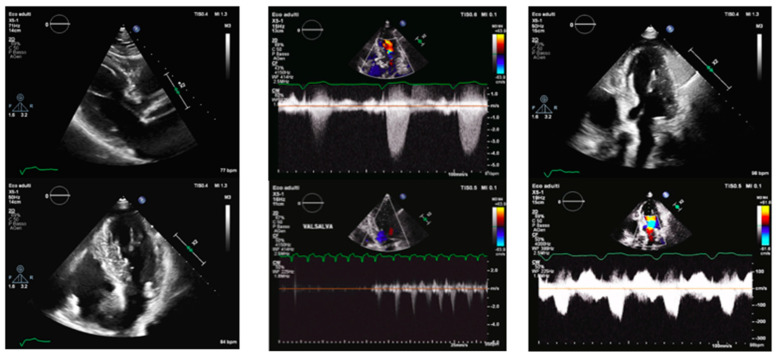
Transthoracic echocardiography of a 79-year-old woman before and after surgical aortic valve replacement and septal myectomy. Left panel. PLAX view (upper) and 4-chamber view (lower). IVS telediastolic diameter = 15 mm; LVEDVi 27 mL/m^2^. Central panel. Aortic valve peak velocity of 4.5 m/s (upper) with a mean gradient of 51 mmHg and dynamic LVOT gradient (lower) of 42 mmHg after the Valsalva maneuver. Right panel: after cardiac surgery. 4-chamber view (upper) and dynamic LVOT gradient of 11 mmHg (lower) after surgical aortic valve replacement. IVS: interventricular septum. LVEDVi: left ventricular end-diastolic volume indexed. LVOT: left ventricular outflow tract.
